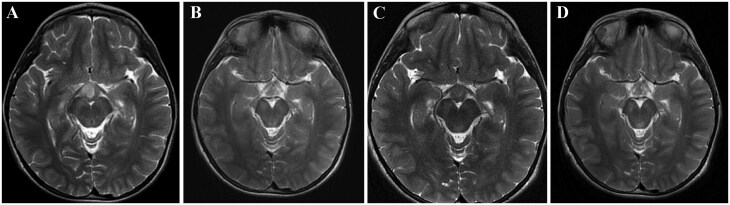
Serial MRI of a patient with NF1-related HH. (A) Initial MRI showing a T2-weighted hyperintense HH in the third ventricular floor. (B) Follow-up MRI after 1 year, demonstrating over 50% reductions in size. (C) MRI taken another year later, showing a slight enlargement of the HH. (D) Subsequent MRI revealing a reduction in HH size once again.
